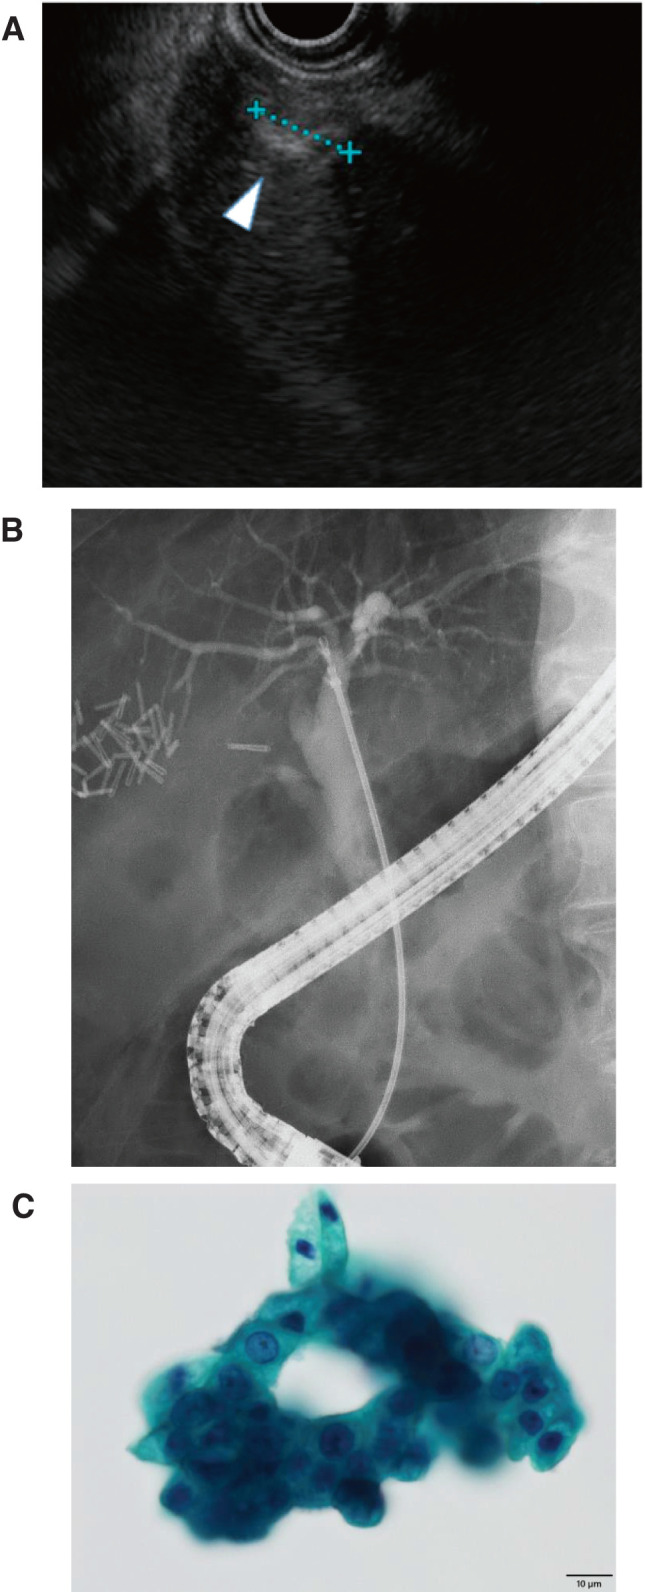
Imaging and cytology before laparoscopic left lateral resection. (A) EUS demonstrating a tumor in segment 3 (white arrowhead), consistent with CT findings. (B) ERCP showing a smooth bile duct without dilation. (C) EUS-FNA sample from the tumor showing adenocarcinoma. Scale bar: 10 μm. EUS, endoscopic ultrasonography; EUS-FNA, endoscopic ultrasonography-guided fine needle aspiration
